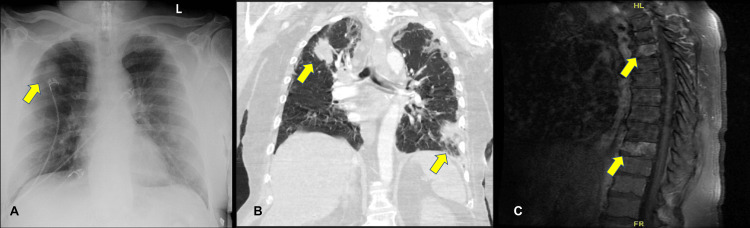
Results of imaging studies done on the patient A. Chest X-ray with a visible mass on the right upper lobe. B. CT scan of the chest. C. MRI of the thoracic spine. CT, computed tomography; MRI, magnetic resonance imaging
